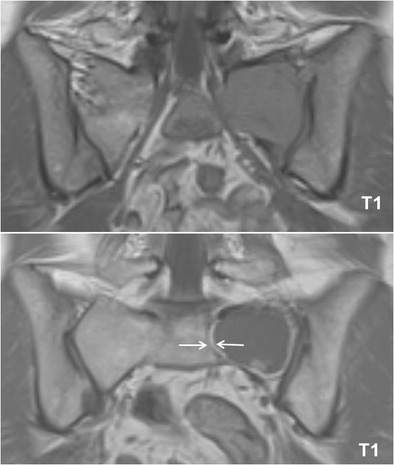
T1-weighted image of the sacrum before (*top*) and after (*bottom*) radiation therapy, presenting the appearance of a peripheral halo of fatty marrow with a characteristic high signal intensity on T1-weighted images (*arrows*), indicating response to therapy
